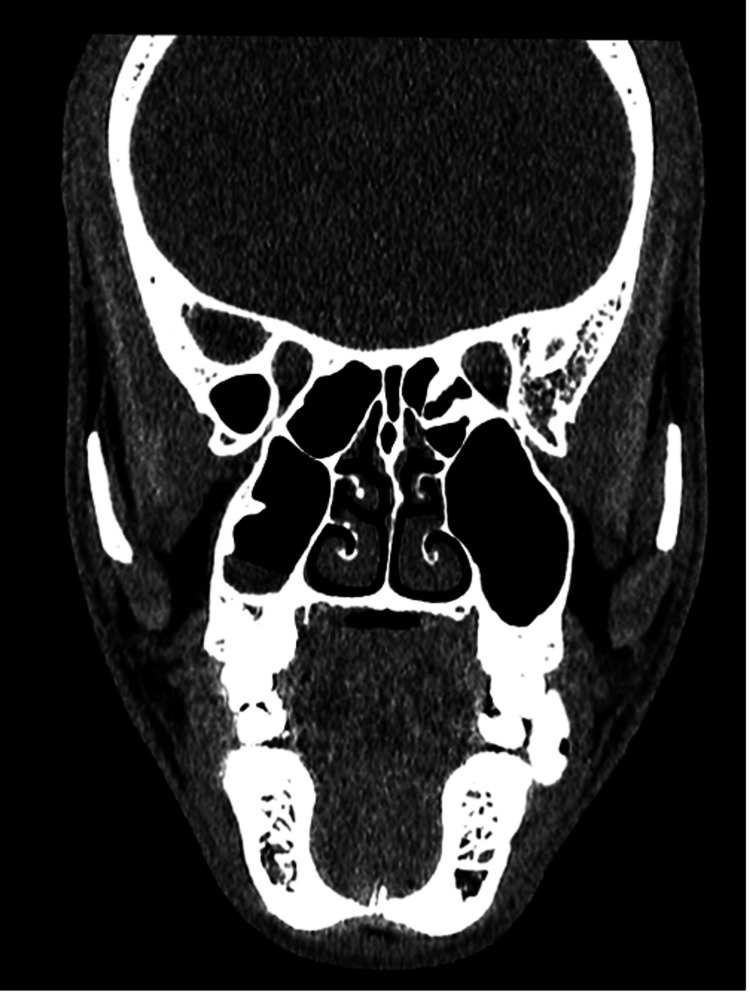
Coronal CT non-contrast image in the soft tissue window showing a circumscribed, ovoid, hyperdense mass with internal gas locule in the inferior left buccal space adjacent to the left lower molar teeth. CT: computed tomography
